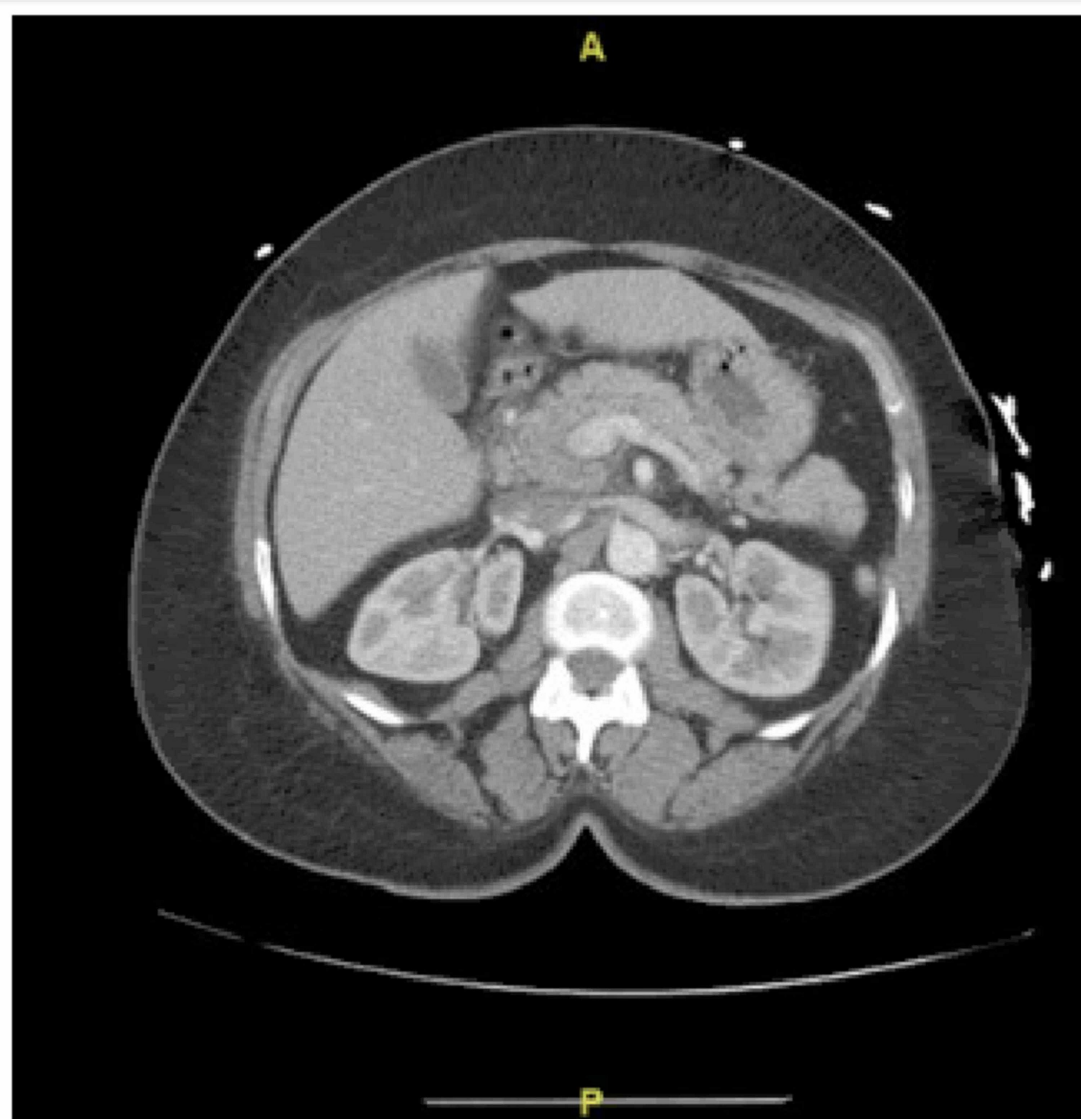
CT scan of the abdomen: mild pancreatitis involving the head and uncinate process, with adjacent edema and prominent lymph nodes.
